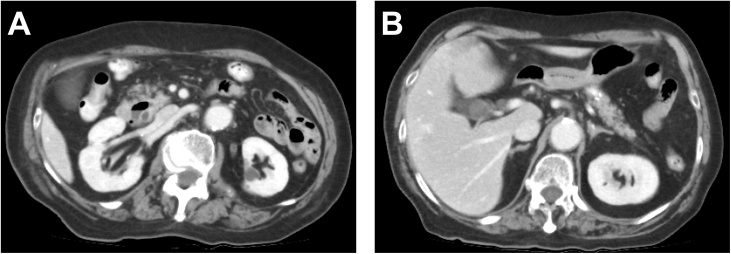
Contrast-enhanced axial CT of the abdomen and pelvis. Contrast-enhanced CT demonstrated a significant improvement of the inflammation in the pancreatic head (A) and body and tail of the pancreas(B).
